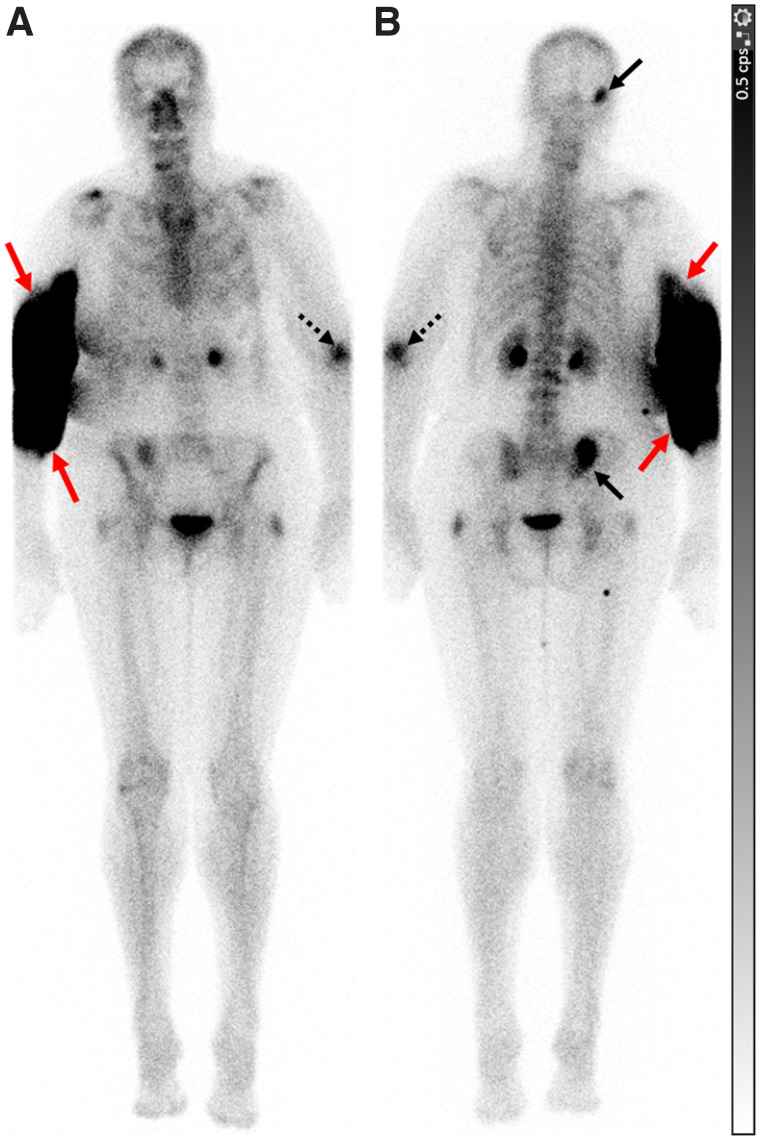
A 68-y-old woman with left breast cancer. WBBS was performed for restaging. Shortly after intravenous injection of 854.7 MBq (23.1 mCi) of ^99m^Tc-MDP in right antecubital fossa, patient complained of swelling and tenderness in her arm. Distal pulses and sensation in right upper extremity were intact. She had infiltration of iodinated contrast medium earlier in day in same arm while undergoing contrast-enhanced CT. She was recommended to use cold compresses and arm elevation at home and had experienced complete recovery when evaluated at next visit (4 wk). WBBS images in anterior (A) and posterior (B) projections show site of RPE around right elbow (red arrows). Patient had metastases to right occipital bone and right iliac bone adjacent to sacroiliac joint (solid black arrows). Prior fracture site in left elbow is also visualized (dashed black arrow).
